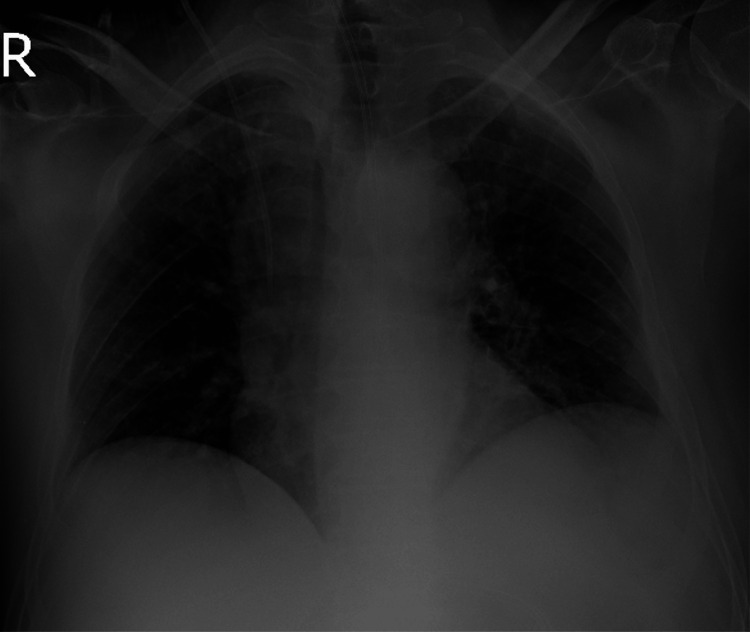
Postoperative X-ray image of the lungs.
